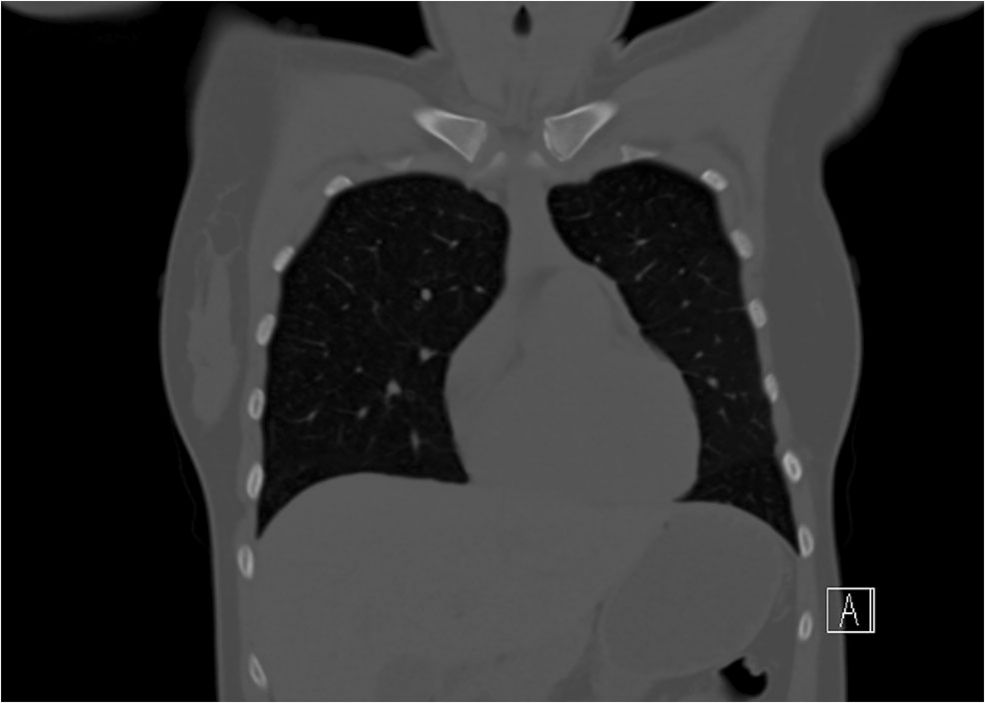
Coronal view of a chest CT using a standard-dose imaging protocol (120 kV/reference 100 mAs; effective dose 6.1 mSv). The ossification stage was classified as 2c
